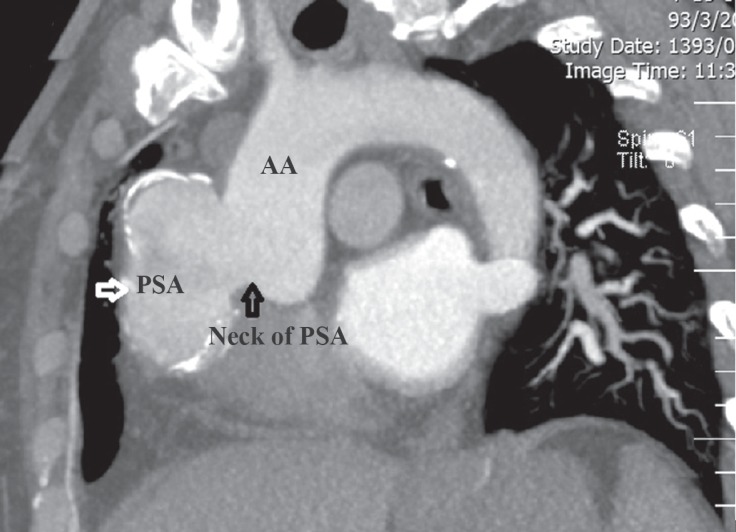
Multidetector computed tomographic angiography before the procedure shows the pseudoaneurysm of the ascending aorta (PSA).
